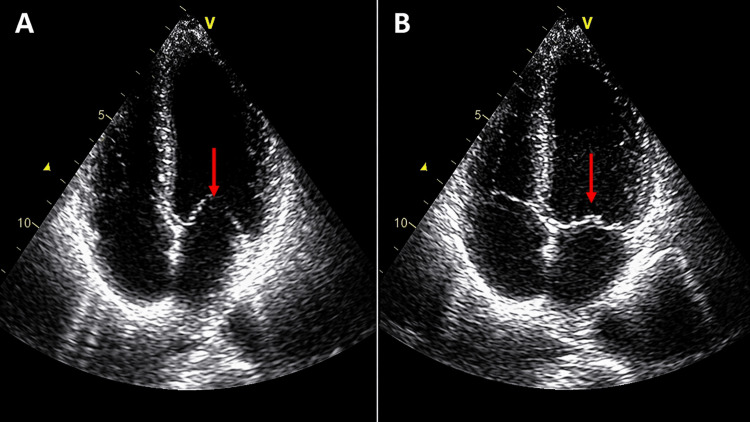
Echocardiographic images (A and B) obtained in systole and diastole, showing normal valve motion without vegetations (arrows)
